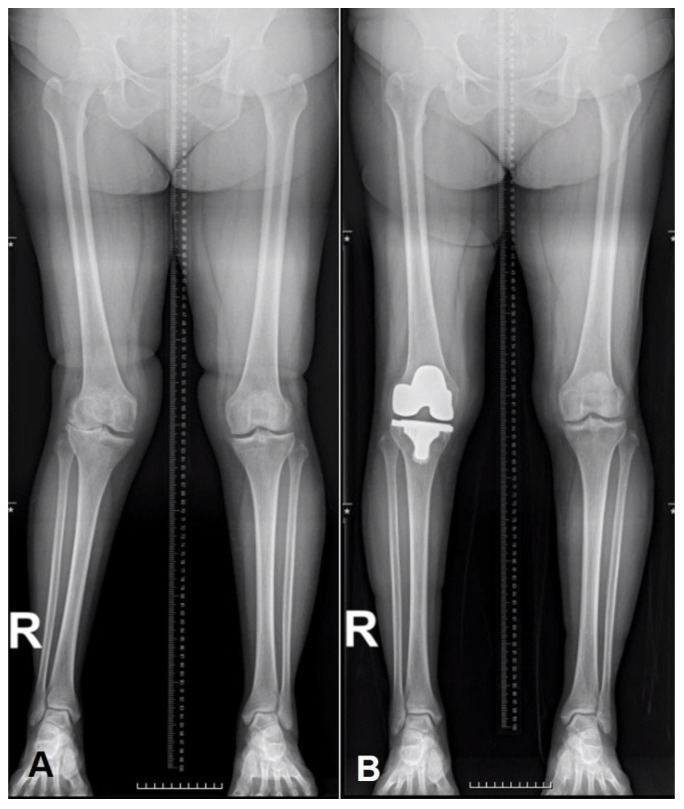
Preoperative and postoperative full-length standing radiographs. Preoperative standing anteroposterior radiograph demonstrating coronal alignment in a knee with lateral compartment OA (A). Postoperative radiograph showing restoration of coronal alignment after TKA using contralateral knee–referenced uKA, while maintaining alignment symmetry with the contralateral knee (B).
